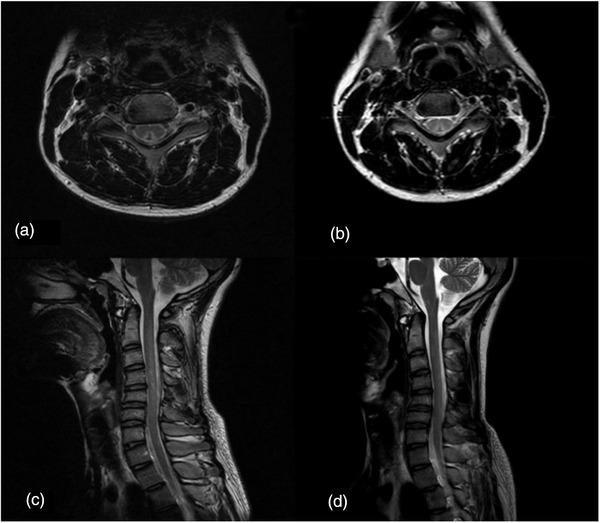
(a, c) Magnetic resonance image (MRI) examination of patients with nitrous oxide abuse at initial consultation showed increased T2 signal in the spinal cord at the C3–C6 level. (b, d) MRI reexamination after 1 month showed reduction of increased T2 signal in the spinal cord at the C3–C6 level
